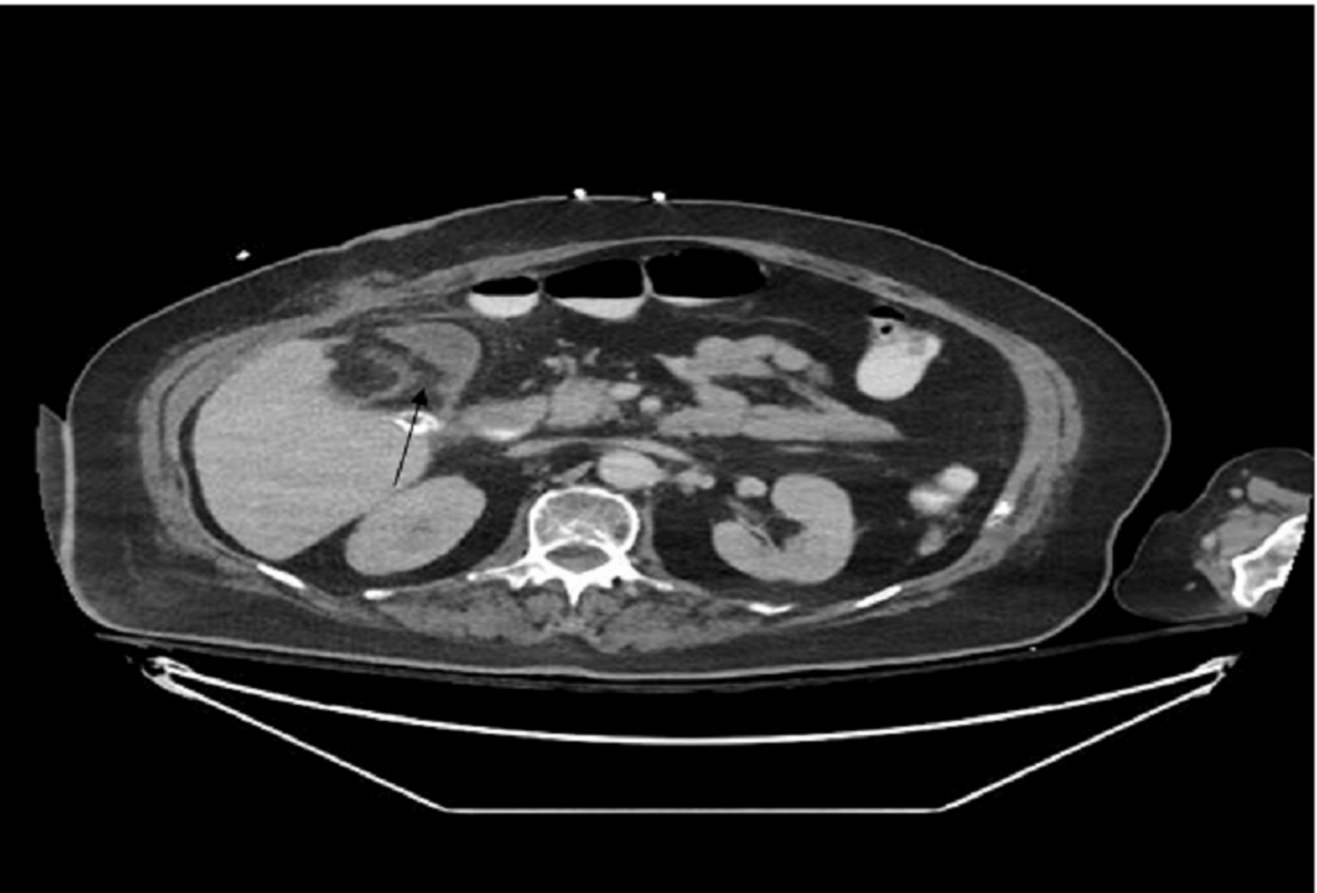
Postoperative CT scan showing findings from the cholecystectomy, including minimal fluid collections in the surrounding area.
